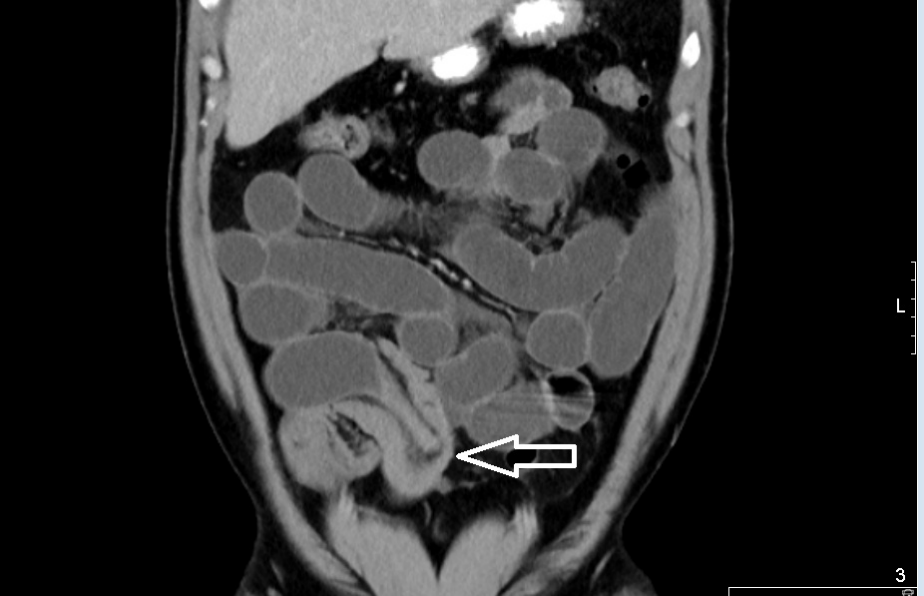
Computed tomography of abdomen - sagittal plane
